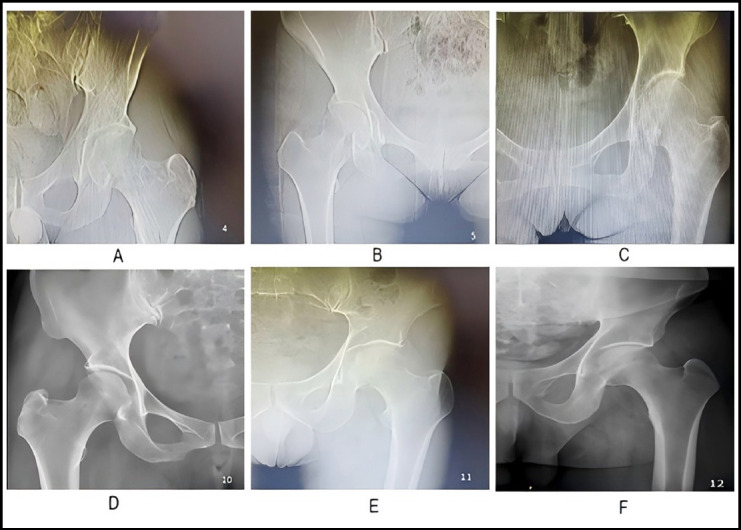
X-Ray findings of aneurysmal bone cyst and femoral neck herniation pit. (A-C) Pathologically confirmed ABCs, showing variable-sized osteolytic lesions of the femoral neck with heterogeneous internal density, partial internal septation, and variable cortical thinning or expansion. (D-F) Femoral neck HPs, showing small, round or oval subcortical radiolucencies with well-defined thin sclerotic margins and generally preserved adjacent cortex.
